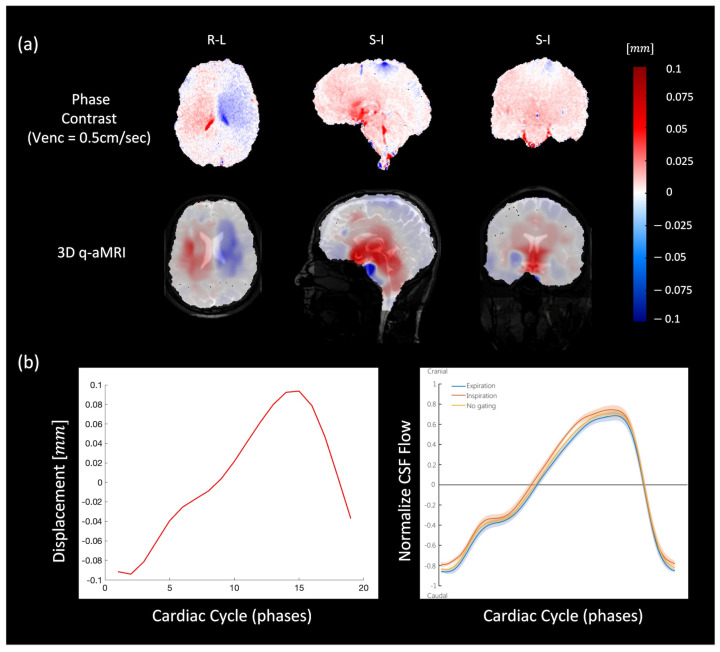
(a) Comparison between PC-MRI (top) and 3D q-aMRI (bottom) for sagittal (S/I direction), coronal (S/I direction), and axial (L/R direction) planes. The estimated field captures the relative brain tissue deformation over time and the physical change in shape of the ventricles by the relative movement of the surrounding tissues. (b) The extracted flow/motion profile through the cerebral aqueduct as extracted by 3D q-aMRI (left), which is comparable to that reported by [46] as shown in the inset (right). Note that [46] the graph seen here is normalized, but the actual CSF flow values reported were an order of magnitude higher than the 3D q-aMRI flow profile.
